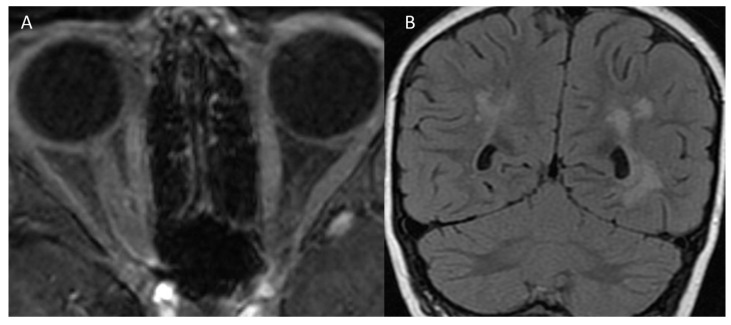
Magnetic resonance imaging scans of case 2. (A) Axial T1 post-gadolinium water selective orbital scan demonstrates enhancement of the right optic nerve. (B) Coronal T2 weighted scan shows multiple bilateral hyperintense white matter lesions in subcortical and periventricular areas of the brain.
